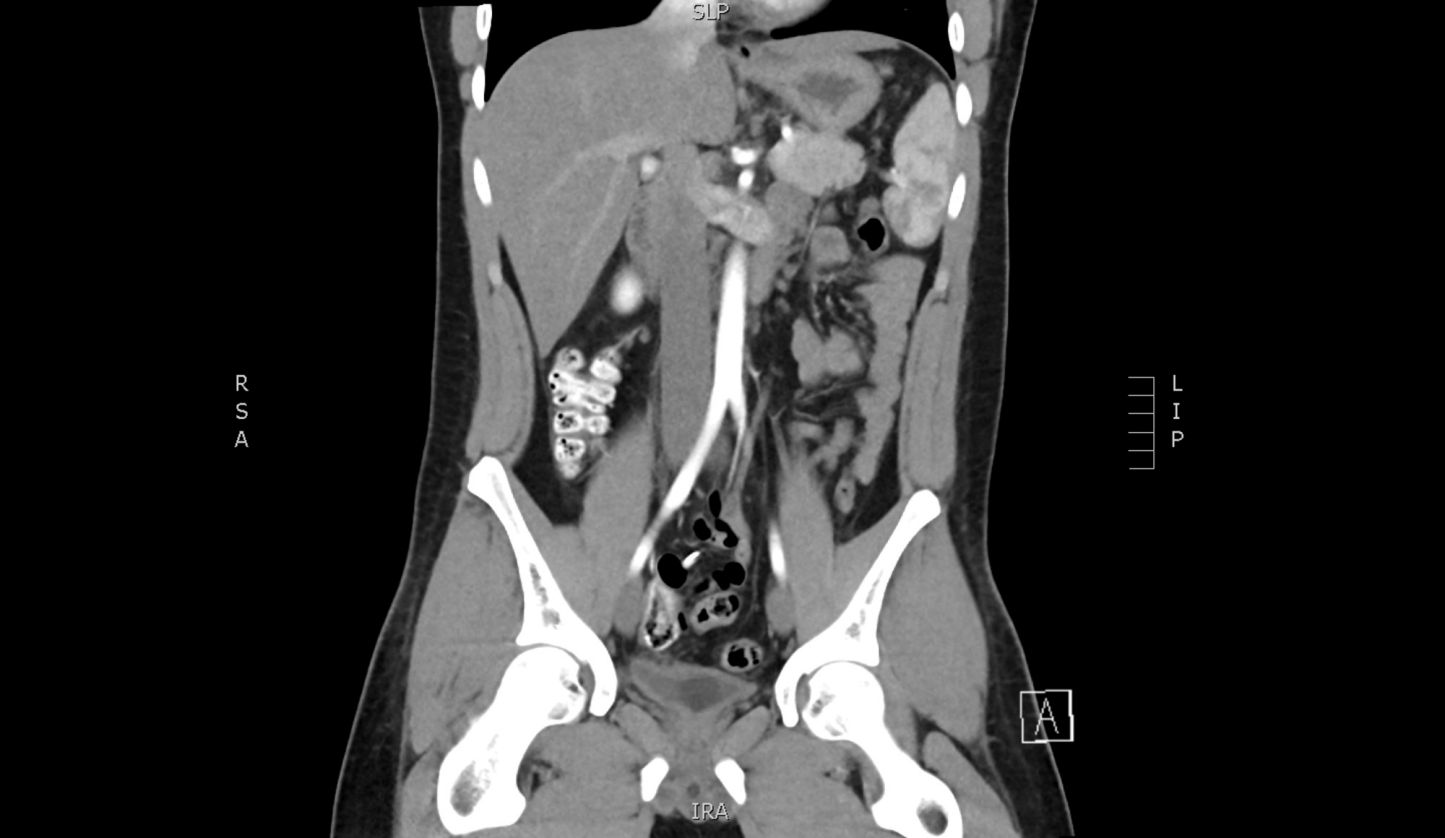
Computed tomographic angiogram on postoperative Day 2 with no blush or haemoperitoneum
